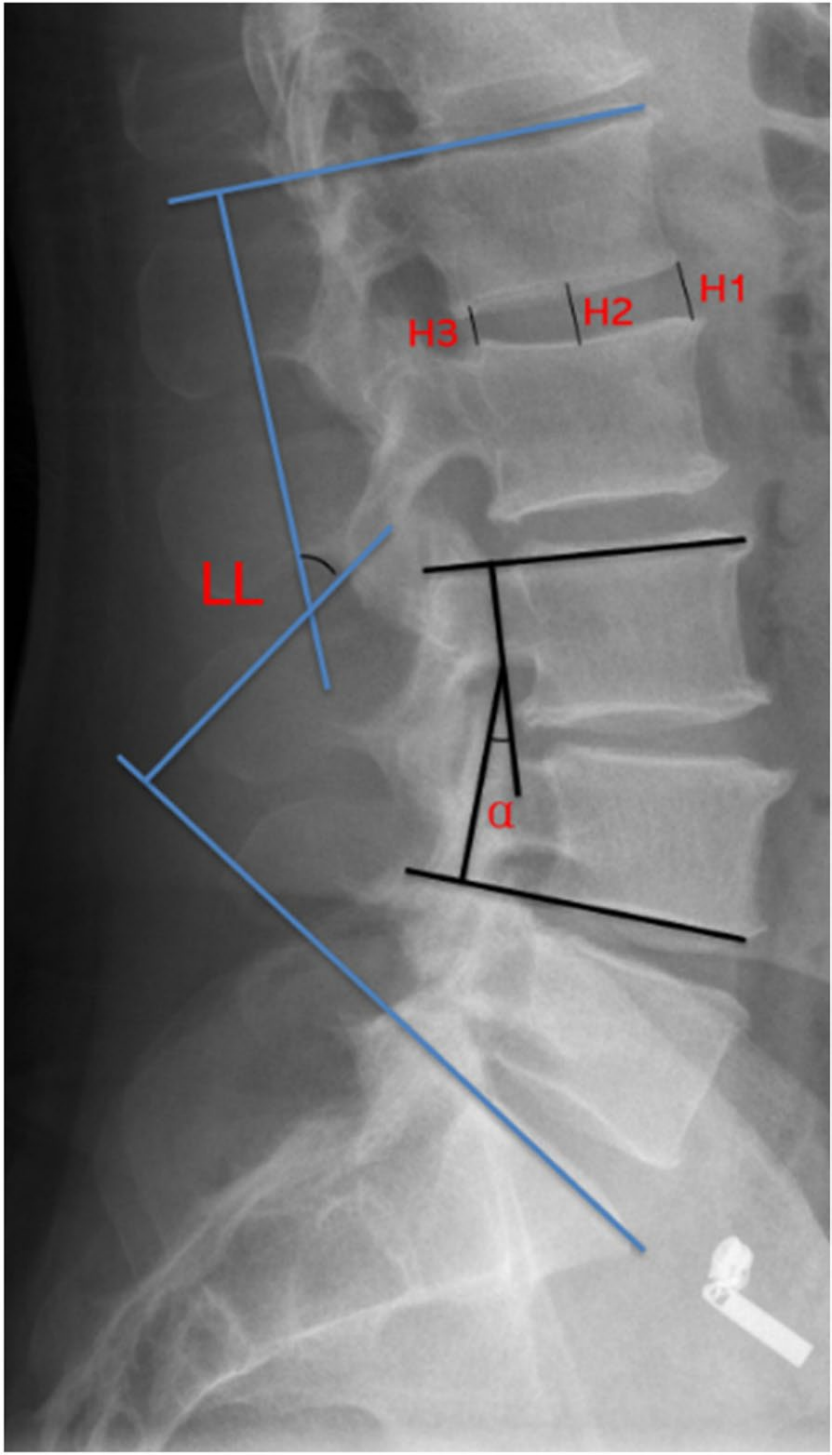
Imaging measurement methods (The range of motion (ROM) of the surgical segment and the adjacent segment is assessed by measured Cobb angle (α) of the surgical segment and adjacent segments on dynamic lateral radiographs. The lumbar lordosis angle (LL) was measured by a standing lateral radiograph. The Cobb angle between upper endplate of L1 and S1 was defined LL
